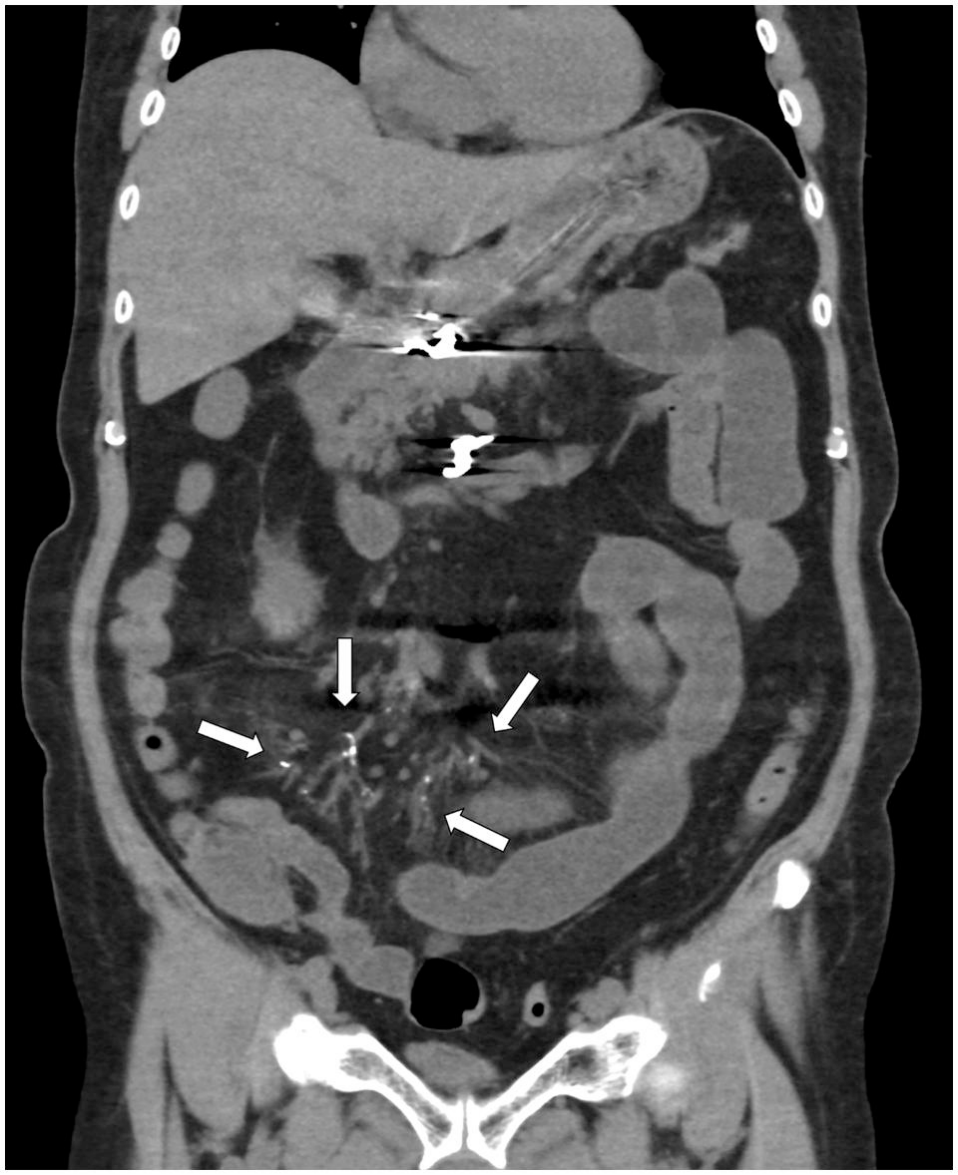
Coronal image of a plain CT scan following transcatheter arterial embolization displaying dot-like high-density areas (arrows) along the distal ileal arteries.
